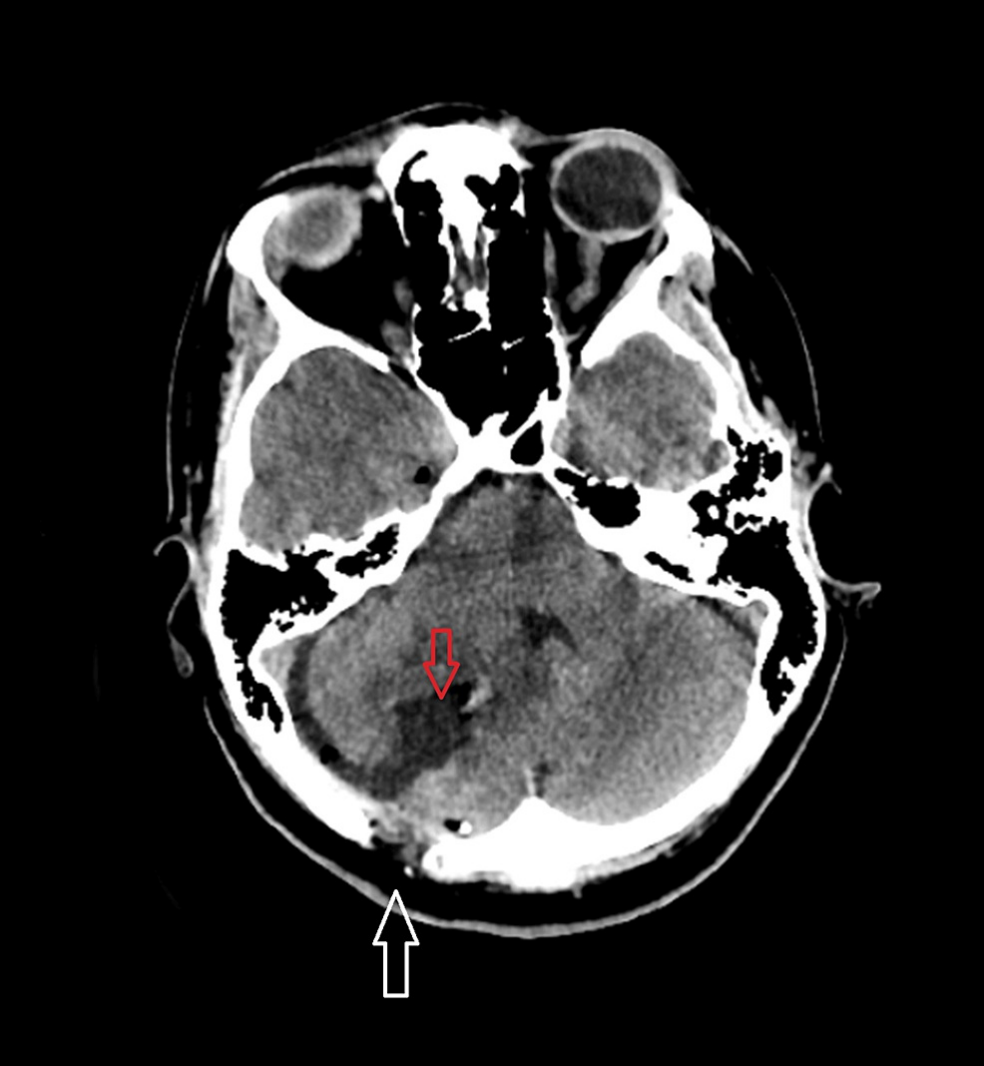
Postoperative computed tomography scan a day after the surgery showing a part of the craniectomy (white arrow) and the area of a visibly totally extirpated tumor (red arrow).
